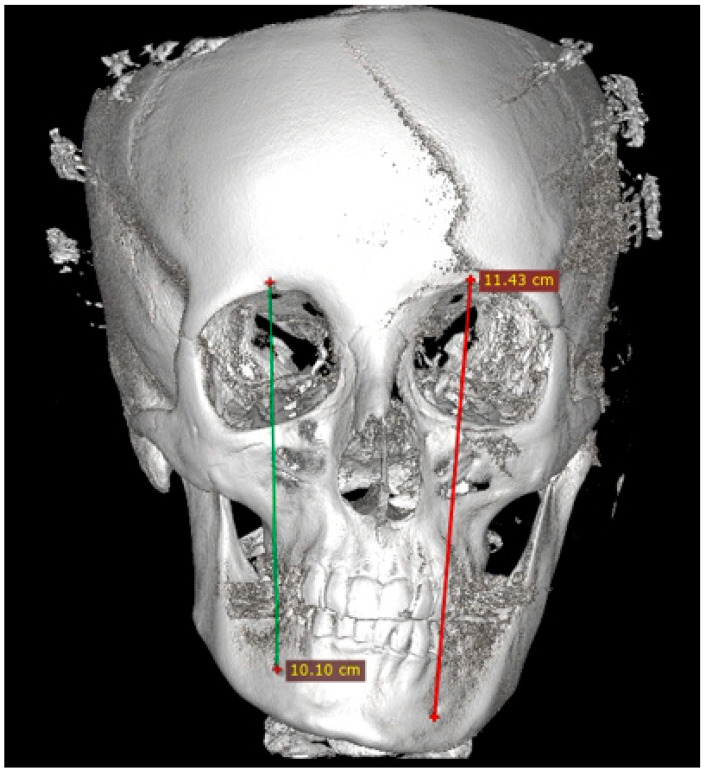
CBCT measurements in maxillo-mandibular asymmetry caused by a right-sided condylar hyperplasia—the measurements in vertical aspect: SOF-MFO. A notable asymmetry and deviations in the foramen position are noticeable.
